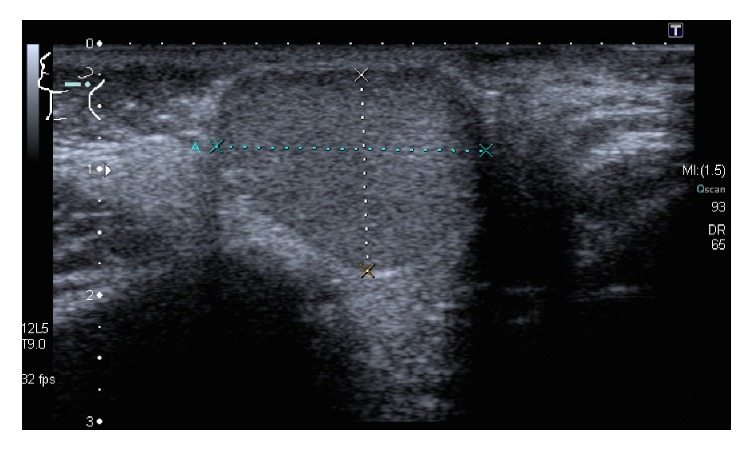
Ultrasonography of the left parotid gland showing a well-defined, homogenic, round, and hypodense mass with a diameter of 2.5 × 2 × 1.9 cm.
